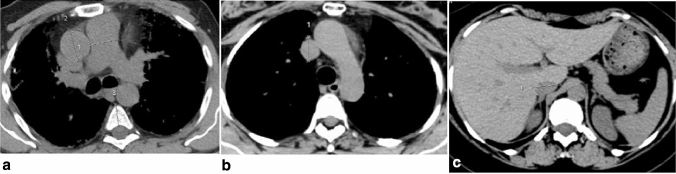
a The evaluated HRCT parameters: diameter of the *main pulmonary artery* (PA), before its bifurcation, and—on the same CT section—diameter of ascending aorta and the mid anteroposterior diameter of the thoracic vertebra. b The evaluated HRCT parameters: diameter of the superior caval vein. 1c: The evaluated HRCT parameters: diameter of the inferior caval vein
